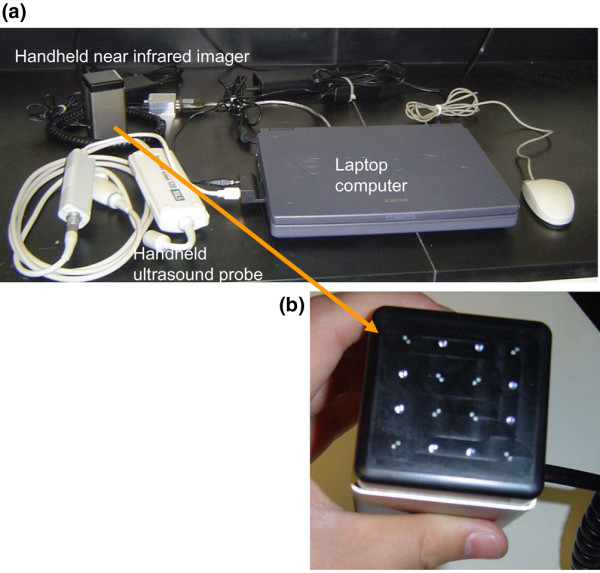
System setup for the dynamic imaging system. (a) System setup for dynamic imaging of suspicious breast lesions: the dynamic imaging system consists of a handheld near-infrared imager, a laptop computer, and a handheld ultrasound probe. (b) Close view of the handheld near-infrared imager.
